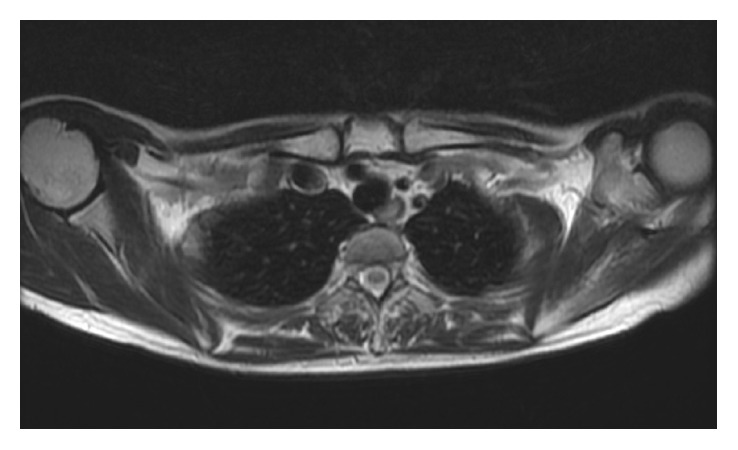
T2-weighted magnetic resonance images taken at the final follow-up 12 years postoperatively. There was no abnormality of the soft tissue or bony structures, although some asymmetry existed.
